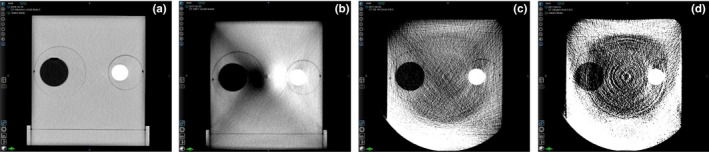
Comparison of images obtained with various techniques under the same viewing conditions (abdomen window/level) in Velocity. (a) Image of original CT scan obtained with the head and neck imaging protocol. (b) Image of CBCT pelvis imaging protocol with beam hardening artifacts. (c) Image of CBCT head imaging protocol with truncation artifacts and increased noise level. (d) Image of CBCT gently imaging protocol with further enhanced noise and artifacts level. CBCT, cone‐beam computed tomography.
